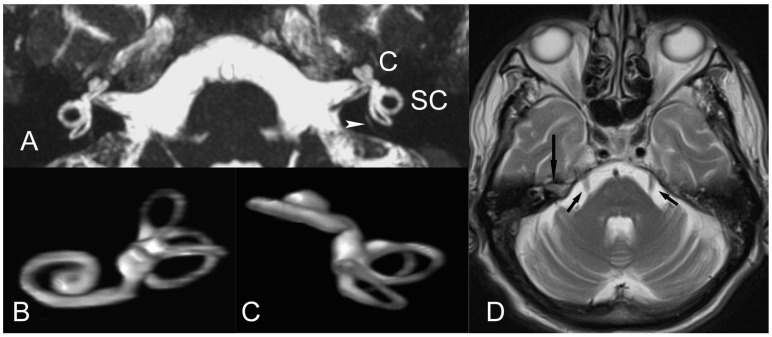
(A) Axial non-gadolinium-enhanced 3D-CISS image (volumetric technique) delineates normal anatomy of the inner ear and the membranous labyrinthine structures on both sides, in a 16-year-old patient with migraine headaches, vertigo, and tinnitus. At this image level, a portion of the fine vestibular aqueduct (arrowhead) is seen. C, cochlea; SC, semicircular canals. (B,C) Three-dimensional-volume rendering (VR) image reconstructions of (A) depict normal, membranous labyrinth. (D) Axial T2-weighted MR image delineates trigeminal nerves (V) (arrows). The vestibulocochlear (auditory) nerve (VIII) also is seen on the right (long arrow).
